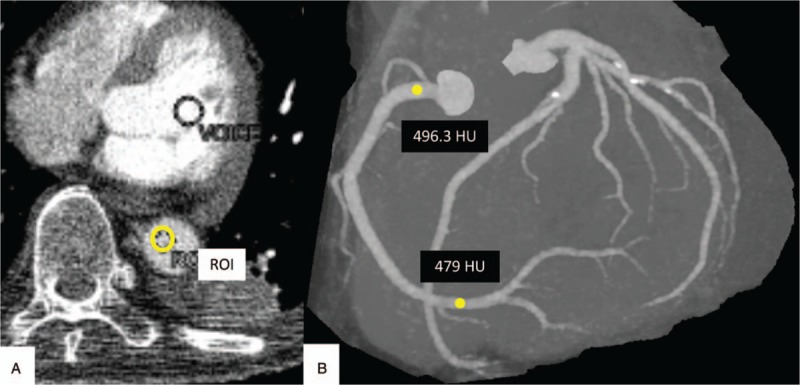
Example case of a 66-year-old man with a ventrally placed ROI. CT values of the proximal and the distal RCA were 496.3 HU and 479.0 HU, respectively. Contrast arrival time was 26.84 seconds. CT = computed tomography, HU = Hounsfield units, RCA = right coronary arteries, ROI = region of interest.
